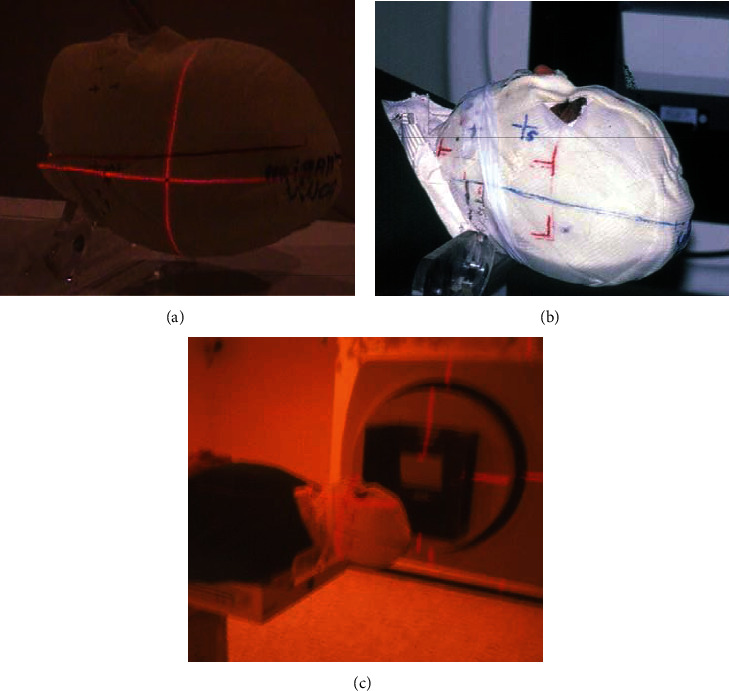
(a) Custom-made rigid mask. (b) Patient wearing the stabilisation mask. (c) 3D treatment planning is done using the CT scan images.
